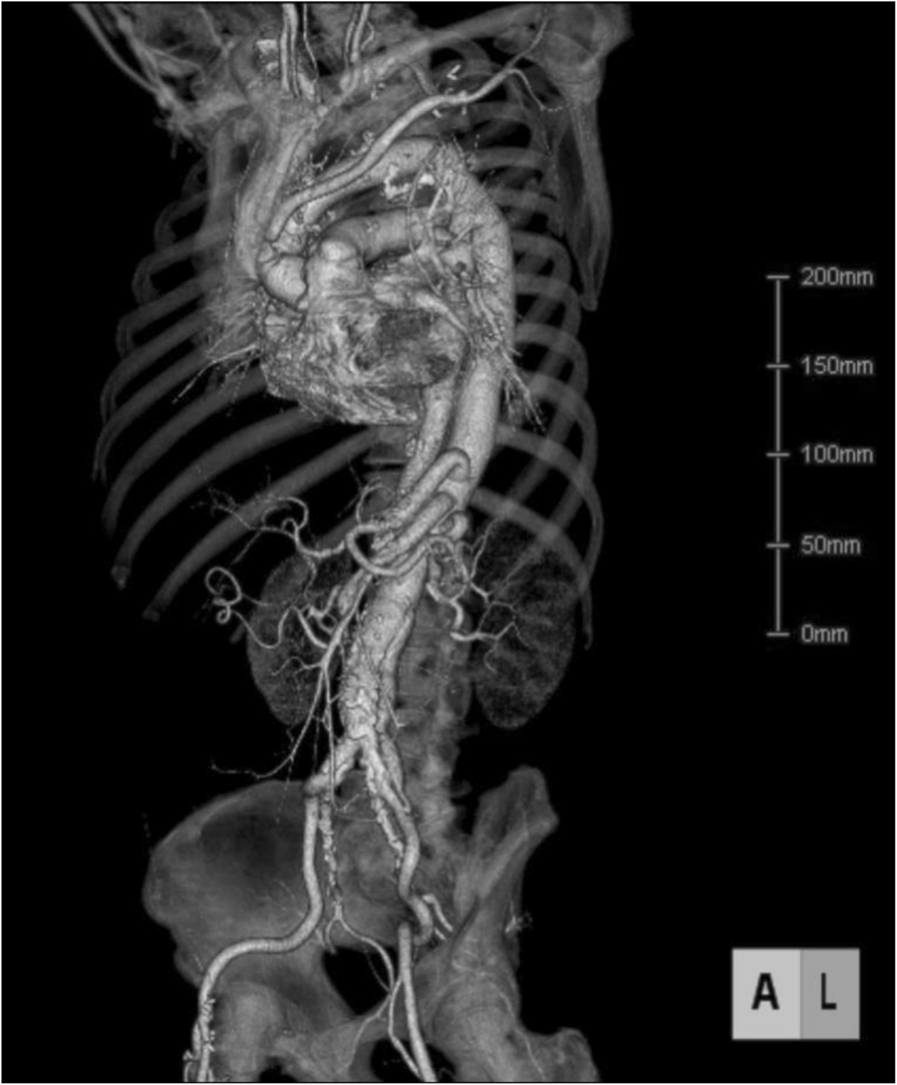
Postoperative CT indicating successful aortic reconstruction.
